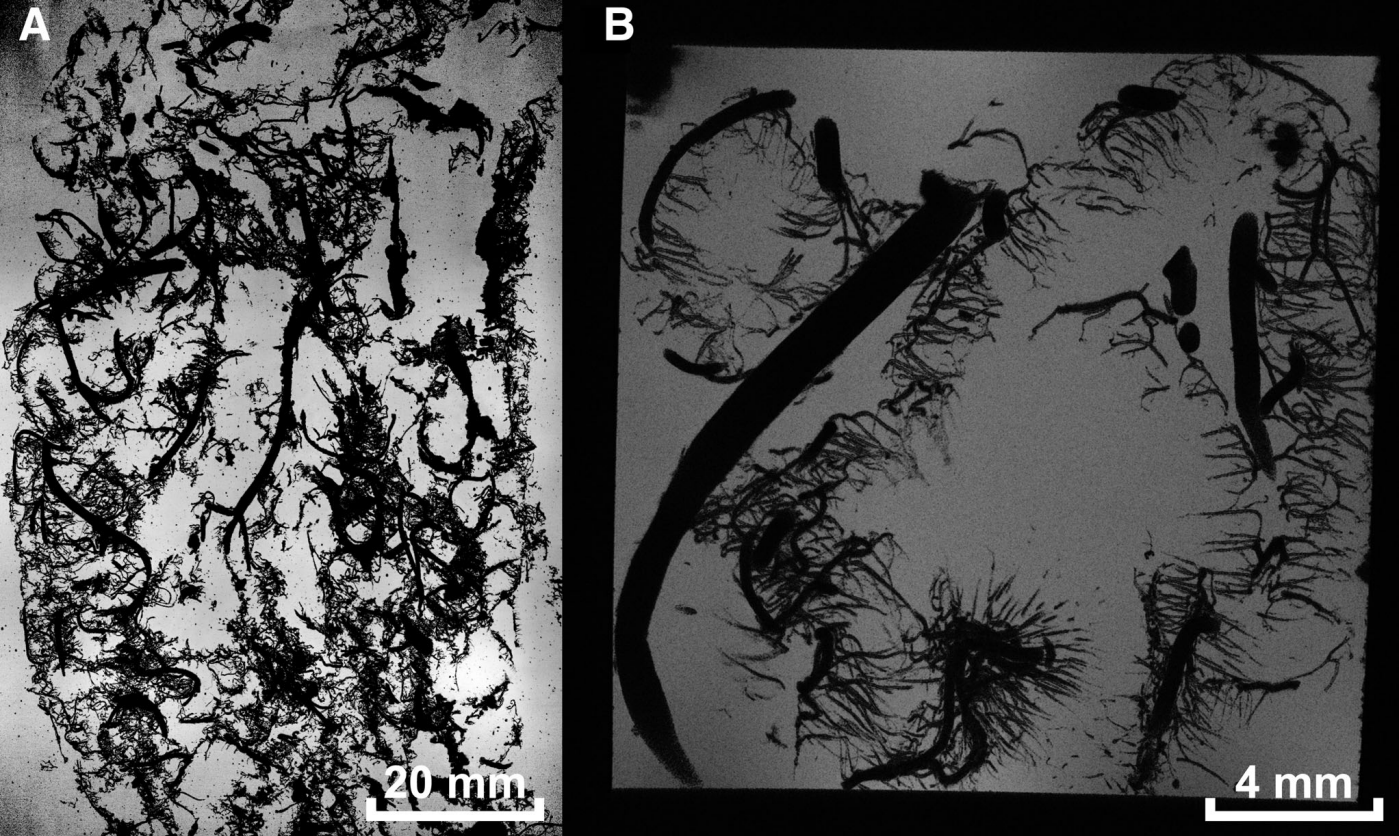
Example MRI data. (A) Minimum intensity projection of 20 slices (1.95 mm total thickness) of 7 T MRI data. (B) Minimum intensity projection of 100 slices (1.50 mm total thickness) of 9.4 T MRI data.
